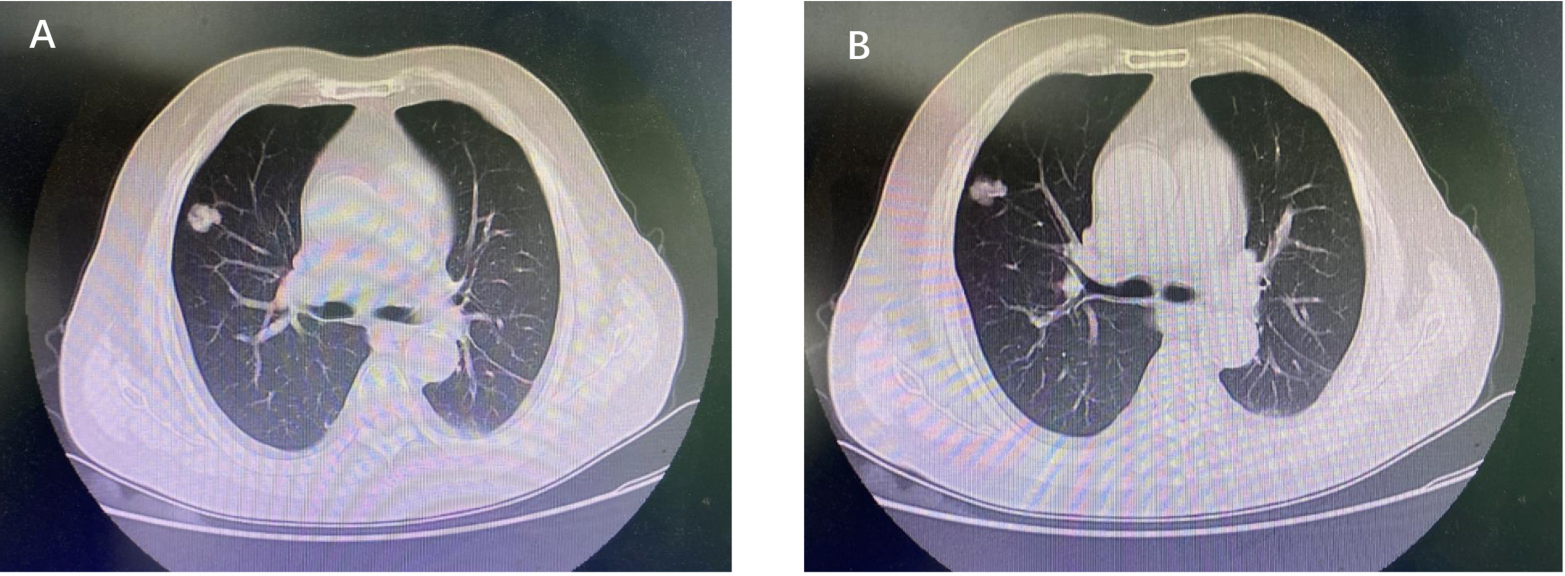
(A, B) Computed tomography of chest revealed a space-occupying lesion in the upper lobe of the right lung.
